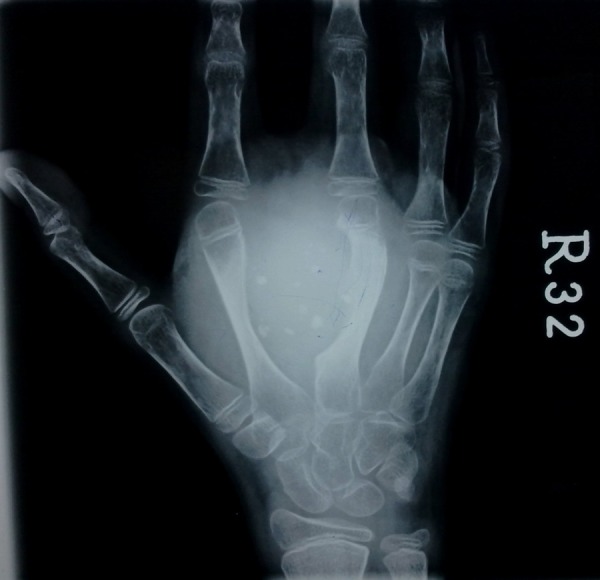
Figure 3: Plain radiograph - right hand: showing soft tissue density swelling with multiple phleboliths.
